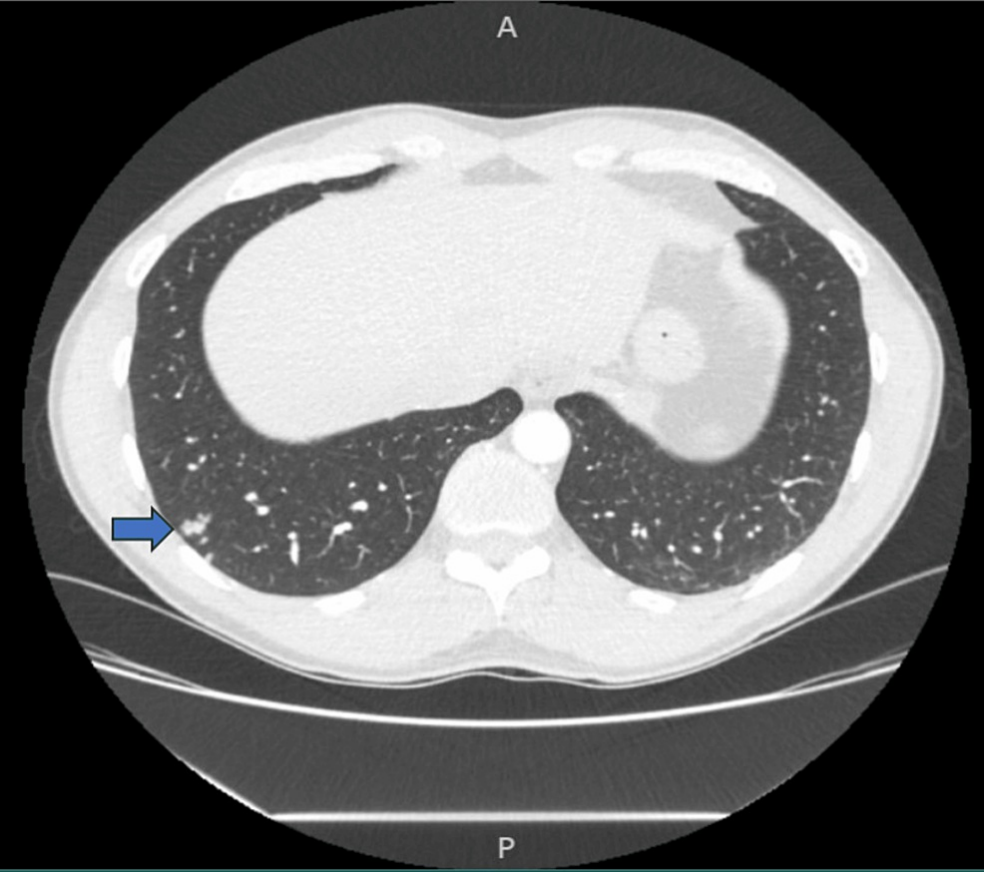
CT chest and abdomen with contrast show few small nodules in the right lower lobe. The blue arrow indicates the area of the small nodules.
